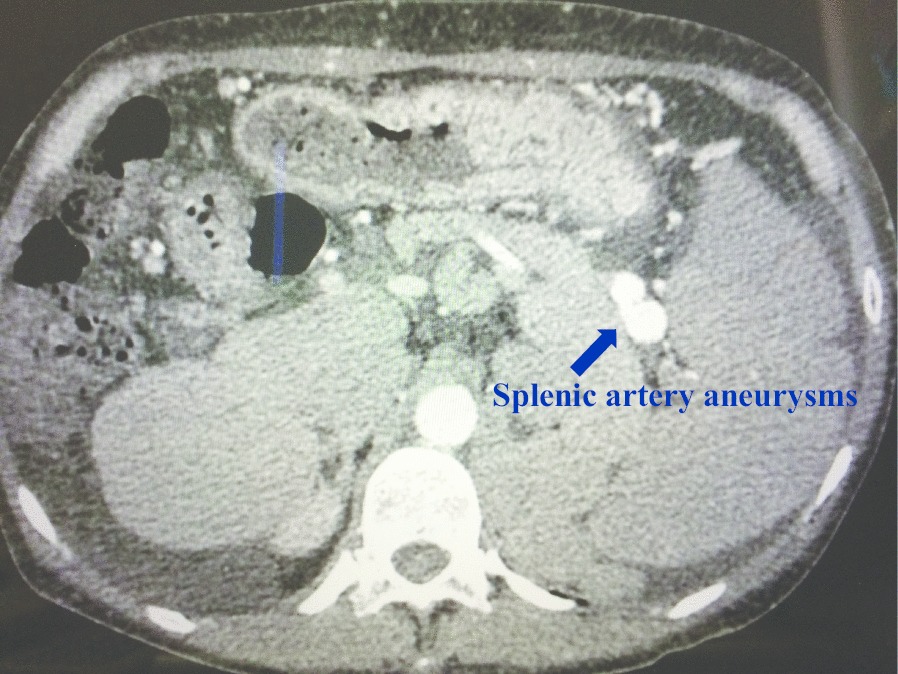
The arterial phase of abdominal dynamic CT. The arterial phase of abdominal dynamic CT reveals the multiple splenic artery aneurysms up to 2 cm in size in the splenic hilum
